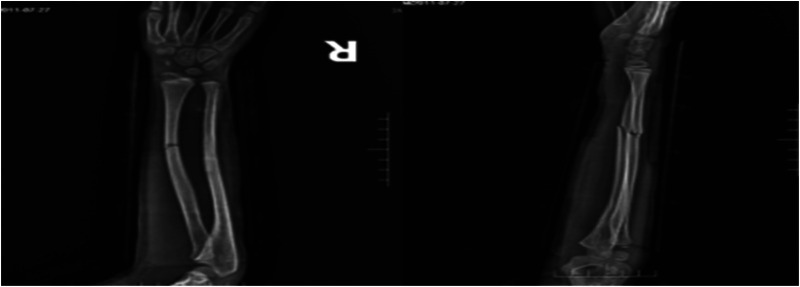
X-rays after manipulation.
